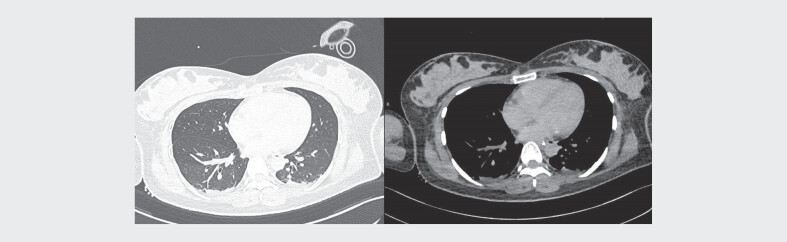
Thoracic computed tomography manifestations 15 hours
